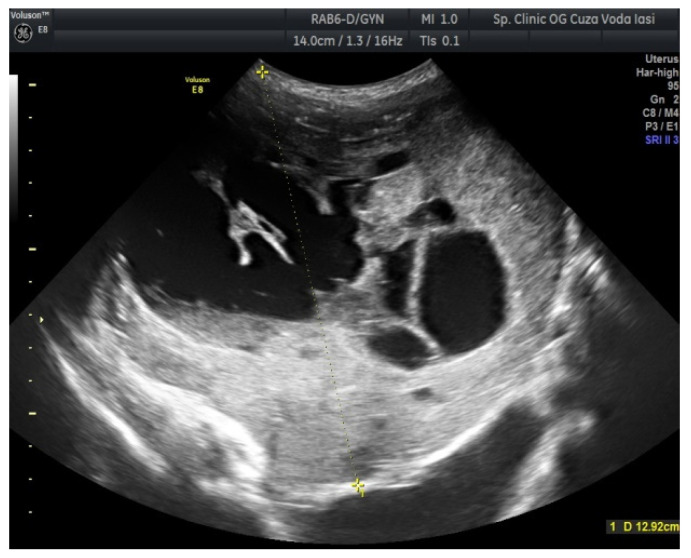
Ovarian ultrasound (US) revealing a large heterogeneous solid mass (right ovary).
